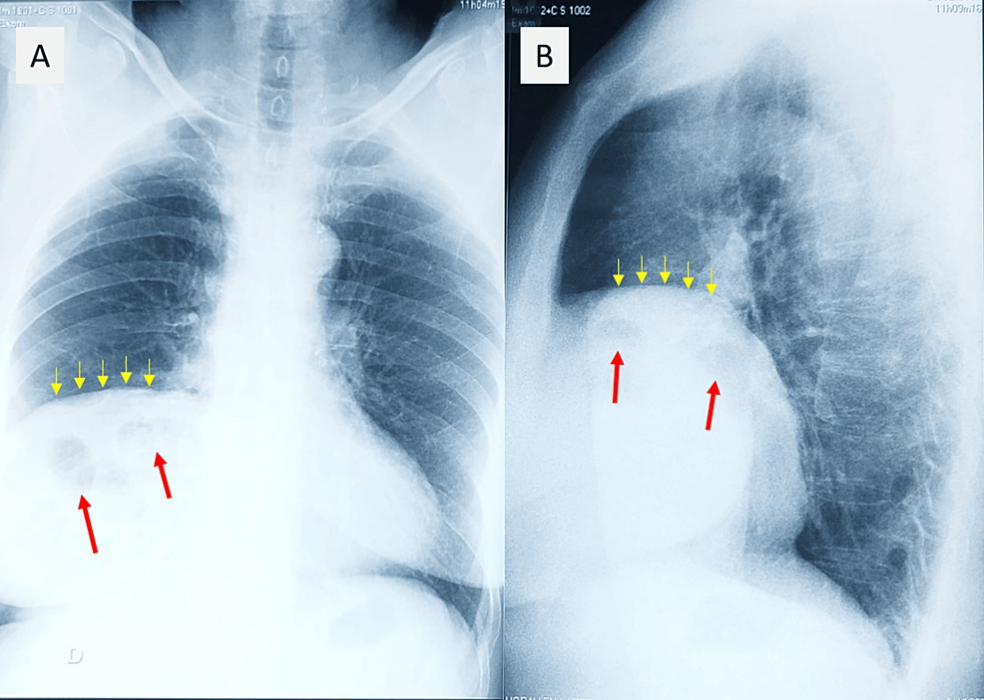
Chest X-ray posteroanterior (A) and lateral (B) views showing an elevation of the right diaphragmatic dome (yellow arrows) with intrathoracic air-fluid images (red arrows).
